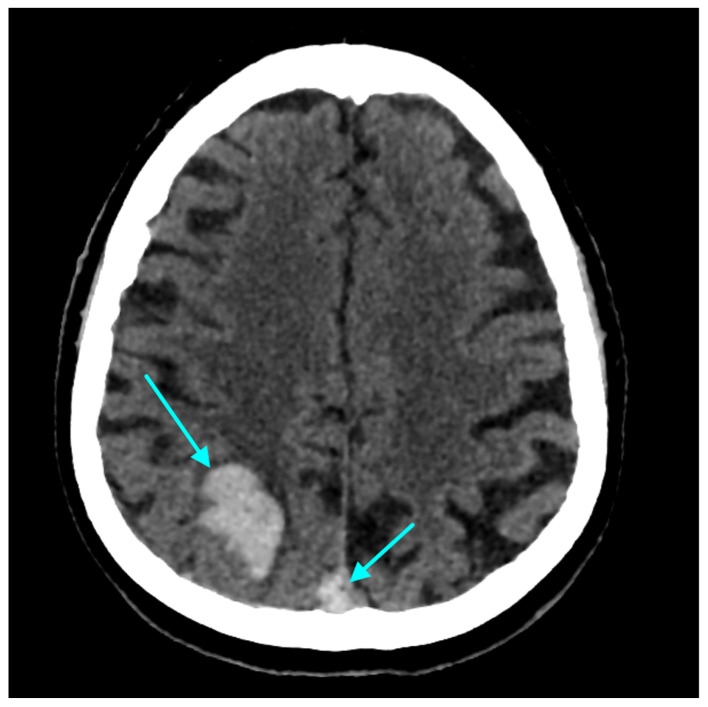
Non-contrast head CT performed in the emergency department in the acute phase demonstrated a hemorrhagic venous infarction in the right parietal lobe, with associated superior sagittal sinus thrombosis (arrows).
